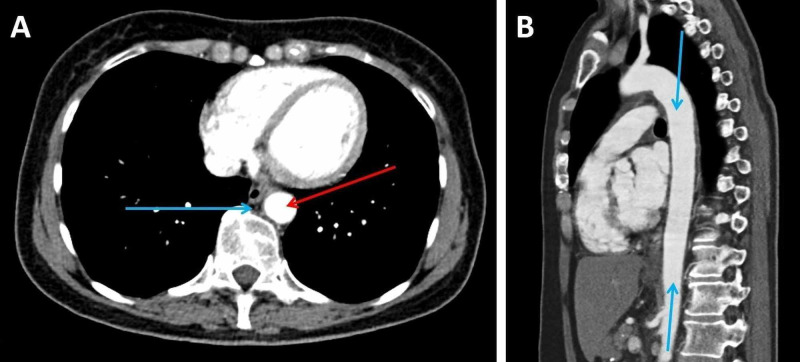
CT imaging of the thorax, abdomen and pelvis performed prior to commencement of chemotherapy (A: transverse plane; B: sagittal plane) A: This image shows a normal posterior mediastinum. The aorta (red arrow) closely abuts the vertebra. The lumen is normal and measures 19mm x 20mm in diameter. The mediastinal fat is barely perceptible and appears normal (blue arrow). B: This image shows a normal calibre thoracic aorta (blue arrows).
